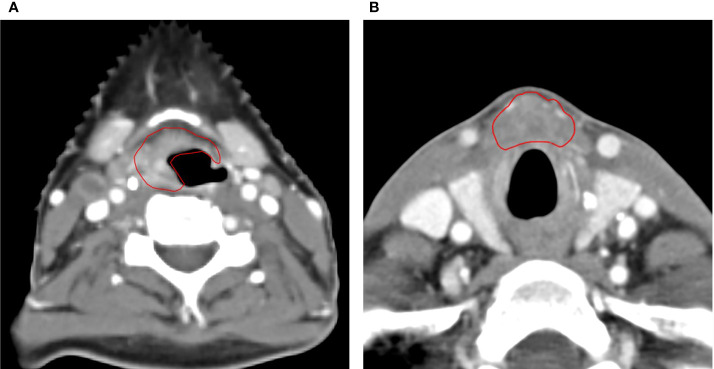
Axial CT scan images of the only patient with a local recurrence outside of the high-dose volume. (A) The primary tumor originating in the vallecula and (B) CT scan of the local recurrence in the prelaryngeal space, approximately 1 cm caudal of the index tumor.
